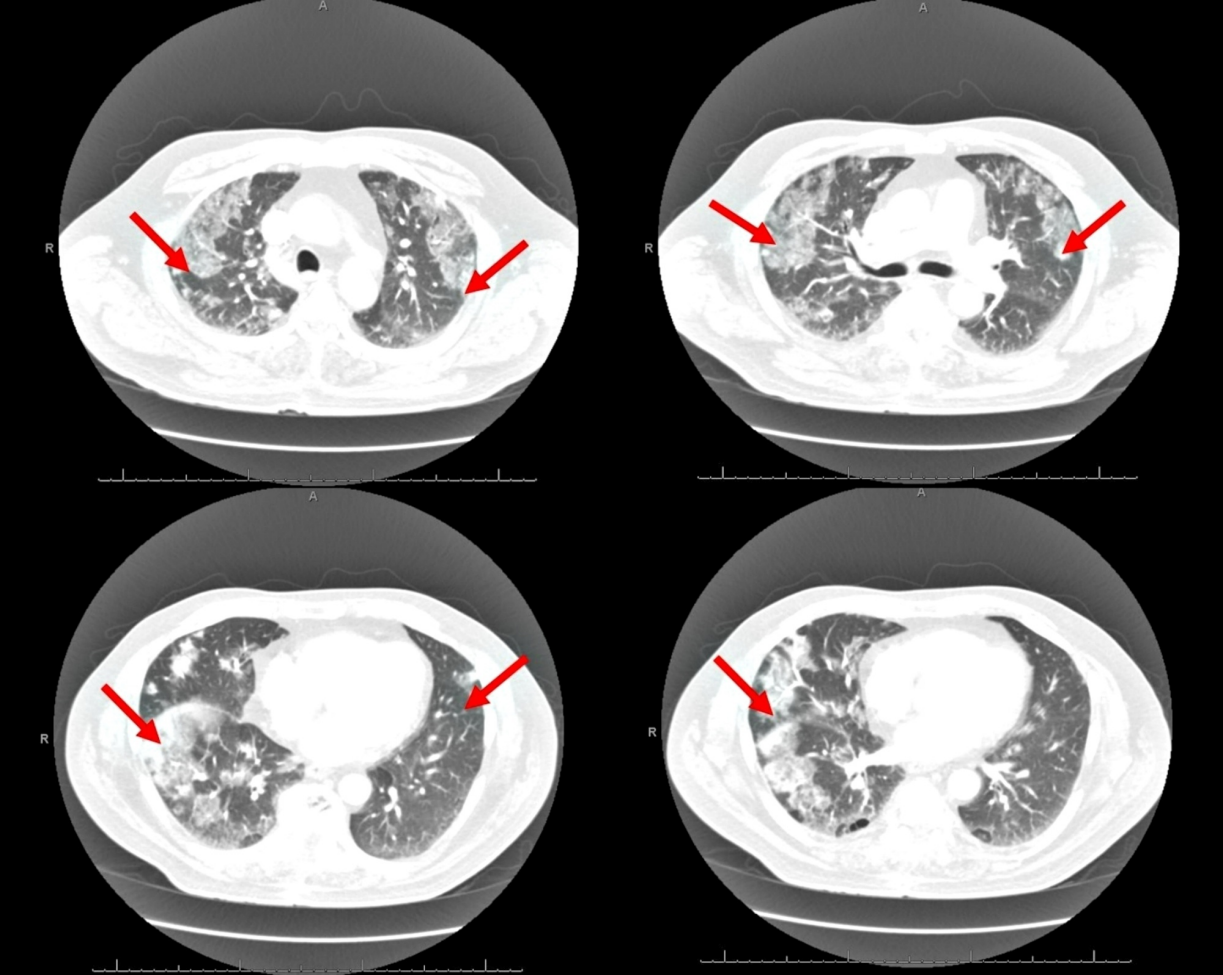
Computerized tomography (CT) scan of chest at presentation showing bilateral pulmonary infiltrates as indicated by red arrows
